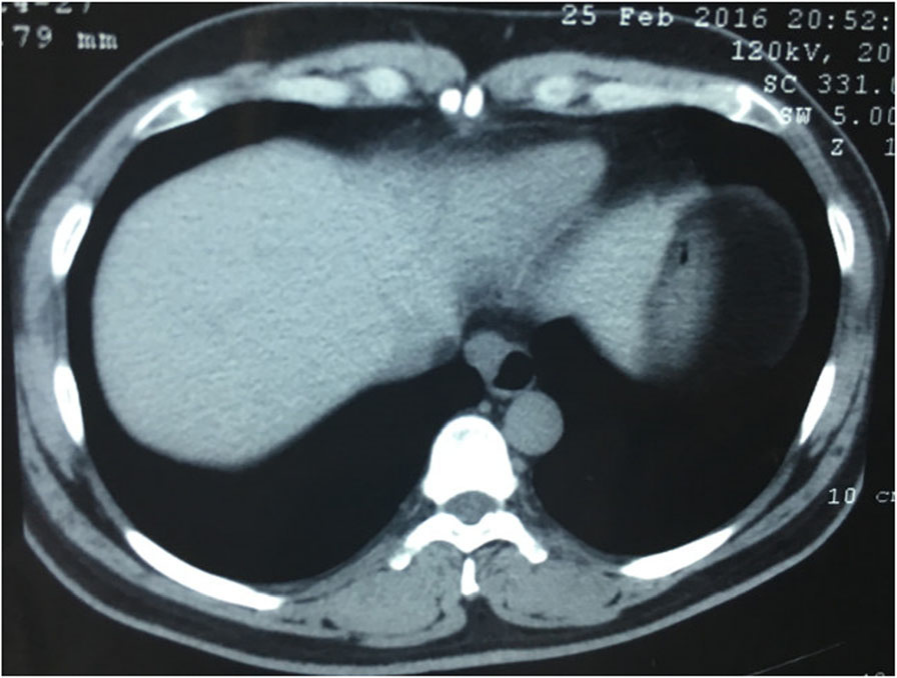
CT demonstrated a soft-tissue attenuation mass from the distal esophageal wall
